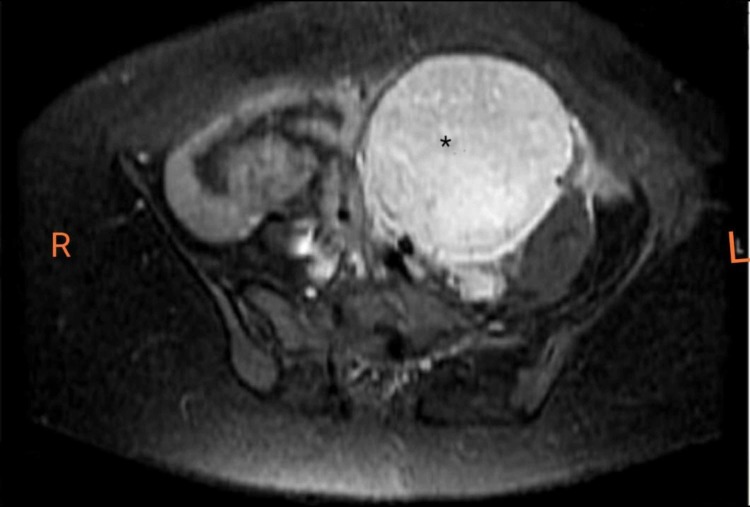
T1-Weighted MRI showing left-sided retro-peritoneal homogeneously enhancing, well-circumscribed mass (asterisk).
